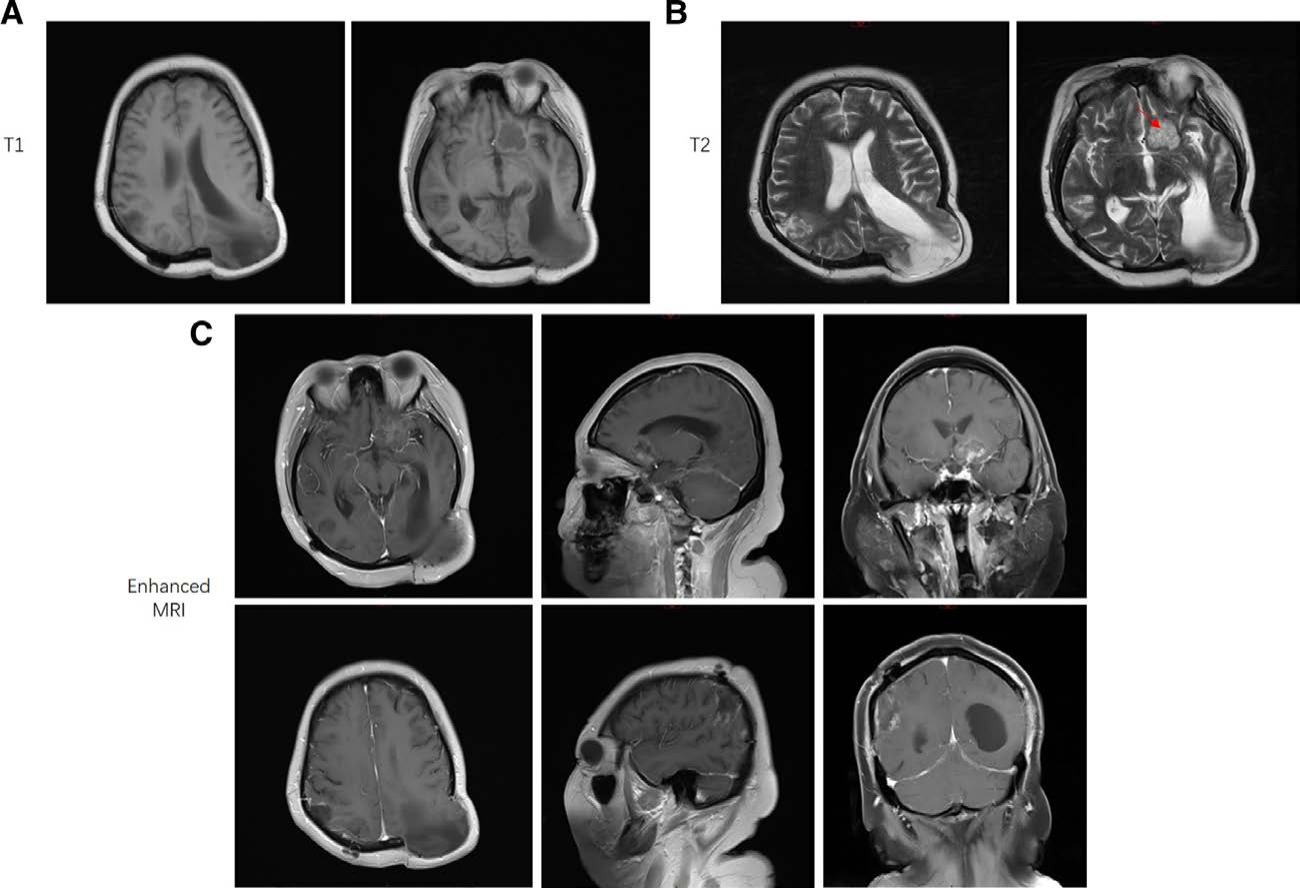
MRI scan of the patient after surgery. The lesion in the right parietal lobe was resected, whereas the lesion in the left frontal lobe (red arrow) was still observed with long signals on T1-weighted (A) and T2-weighted (B) images. Enhanced MRI scanning showed that the lesion was only mildly enhanced after treatment (C).
